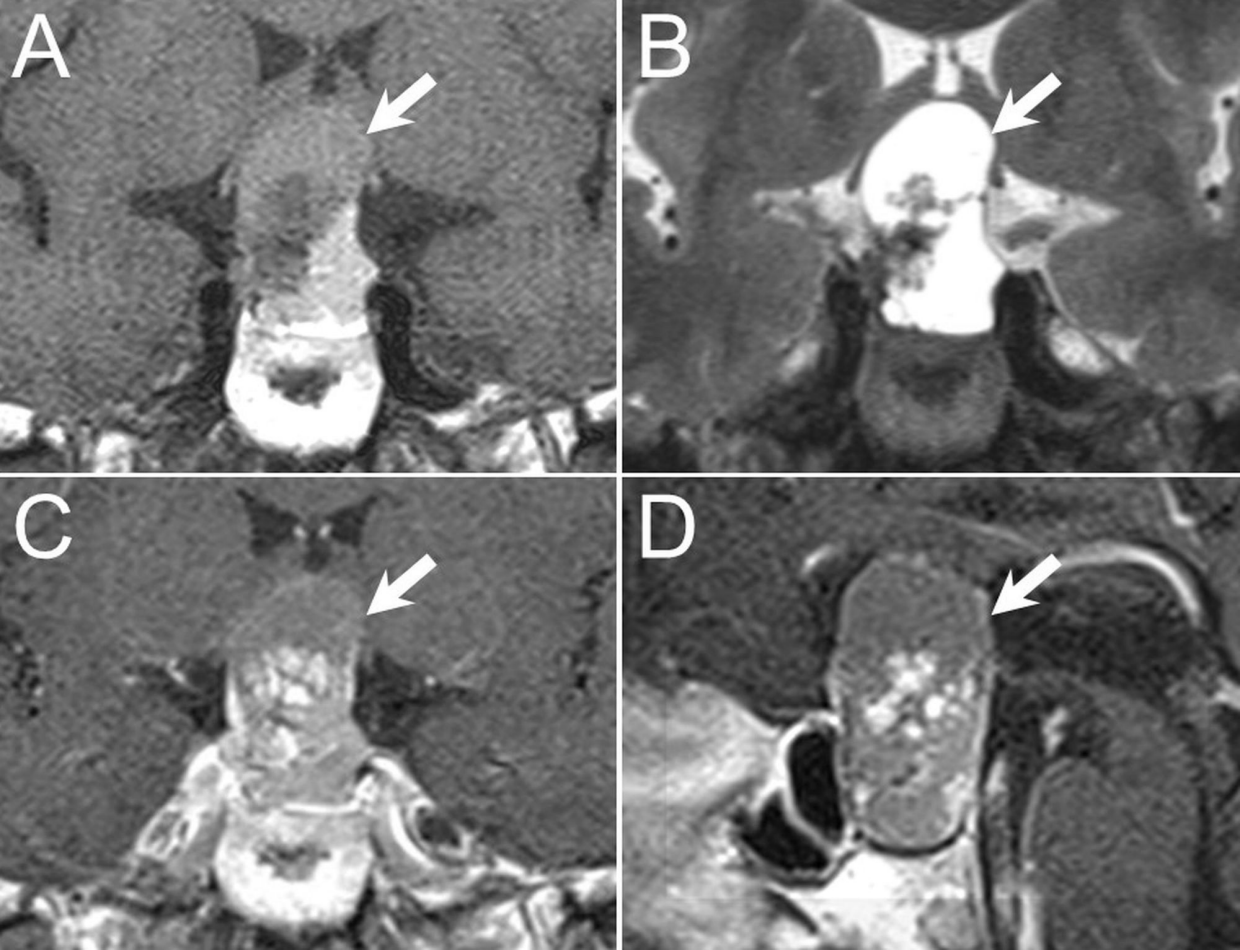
Pre-operative magnetic resonance imaging (MRI) Pre-operative MRI image revealed a mass lesion (arrows) over sellar region with upward optic nerve compression, presenting as a heterogeneous signal change over T1-weighted image (A: coronal view), and a low signal intensity in the central part surrounded peripheral high signal over T2-weighted image (B: coronal view). After gadolinium contrast injection, heterogeneous enhancement was noted over coronal (C) and sagittal (D) views.
